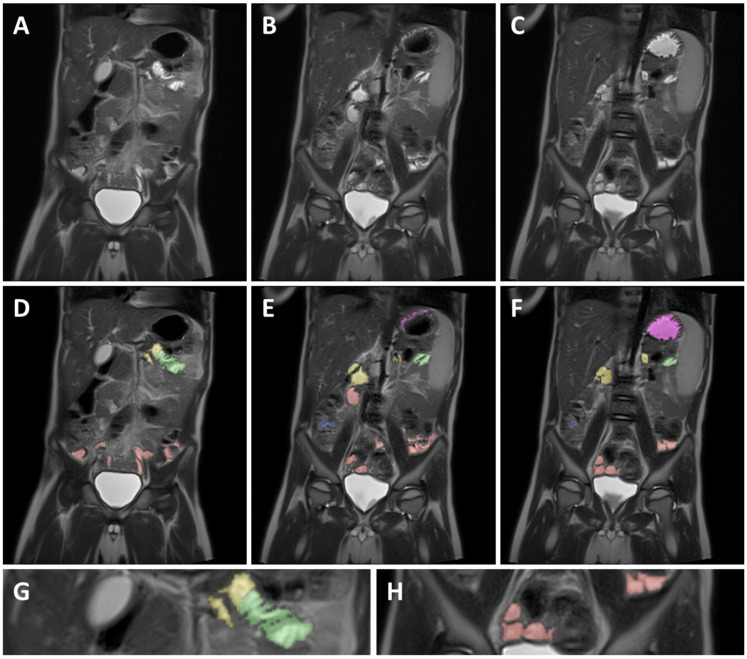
Example of MRI identification of fluid pockets in different GI segments on 3 coronal T2 weighted spin echo images of a 5-year-old child. (A–C) are unmarked images; (D–F) are the corresponding marked images. Purple marked areas are located in the stomach, yellow in the duodenum, green in the jejunum, red in the ileum, and blue in the colon. (G) is a detailed view of the jejunum (green), showing the circular folds as the typical striped pattern in the fluid pocket. (H) is a detailed view of the ileum marked in red, showing the smooth intestinal wall.
